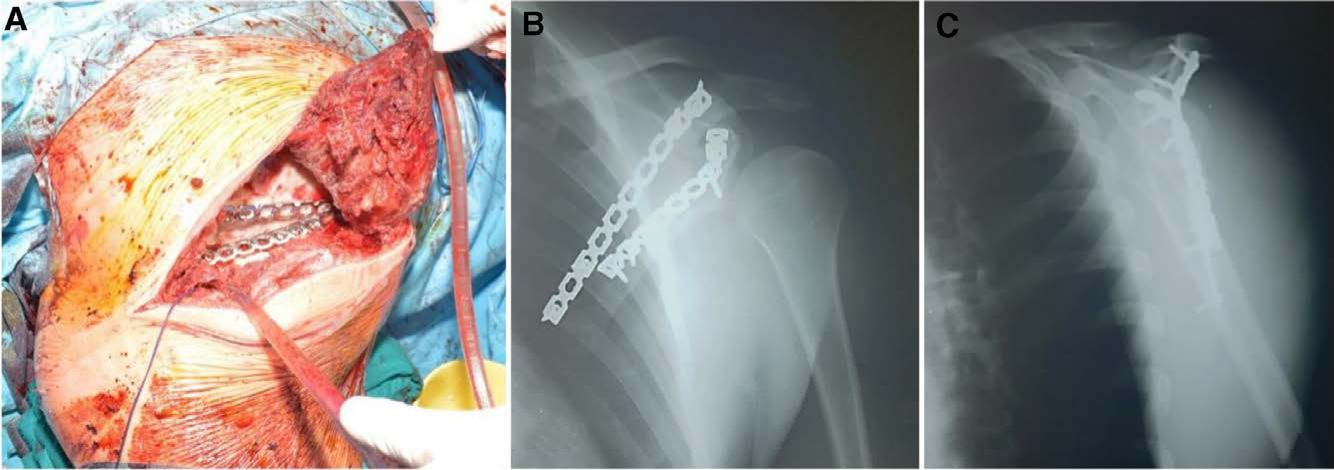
(A) Intraoperative aspect of plate positioning, postoperative X-ray (anteroposterior view – B) of the shoulder and lateral view (C).
